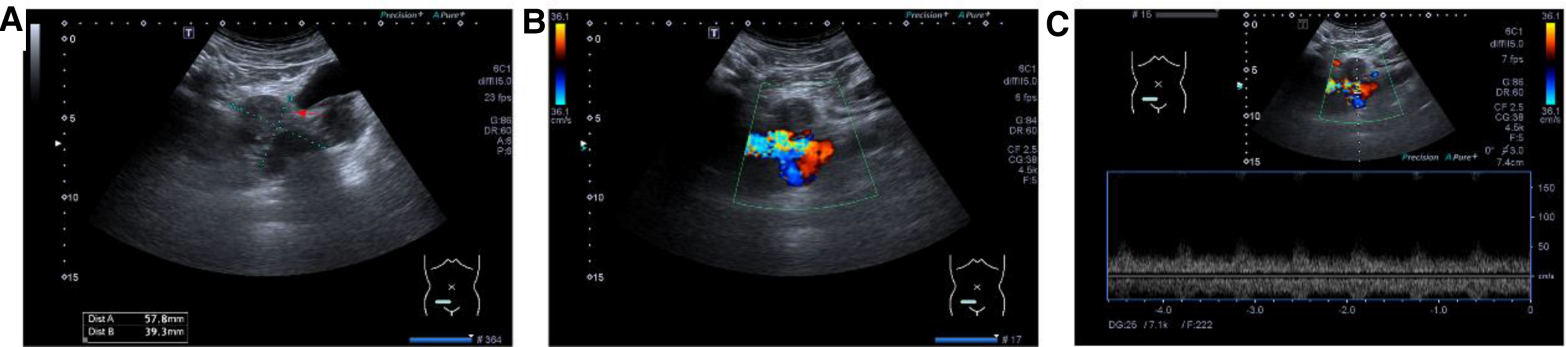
(A) Part of the irregularly shaped anechoic lesions became hypoechoic (red arrow) in the B-mode ultrasound. (B) Color Doppler flow imaging showed the colored mosaic pattern decreased in volume. (C) Spectral Doppler showed the systolic blood flow velocity is reduced after embolization.
